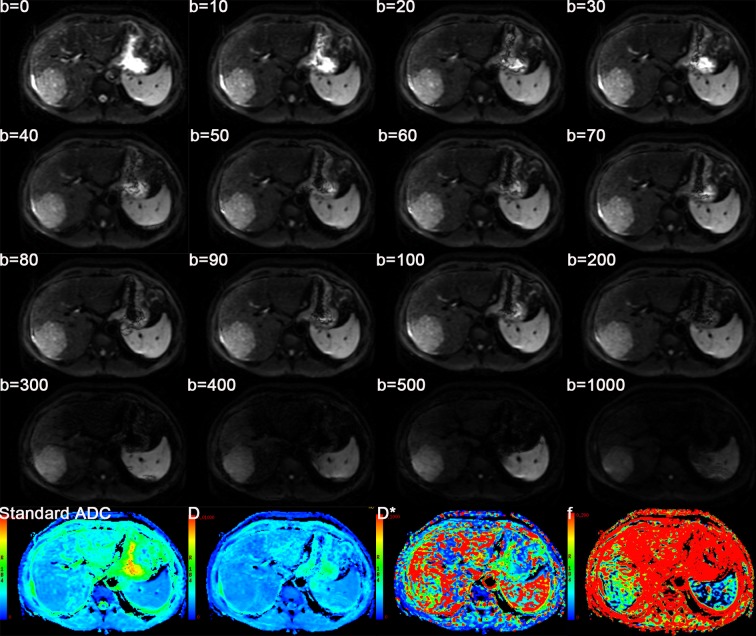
Axial MR image from the 16 b IVIM DWI sequence and IVIM-derived parameters maps of Standard ADC, D, D* and *f*.
